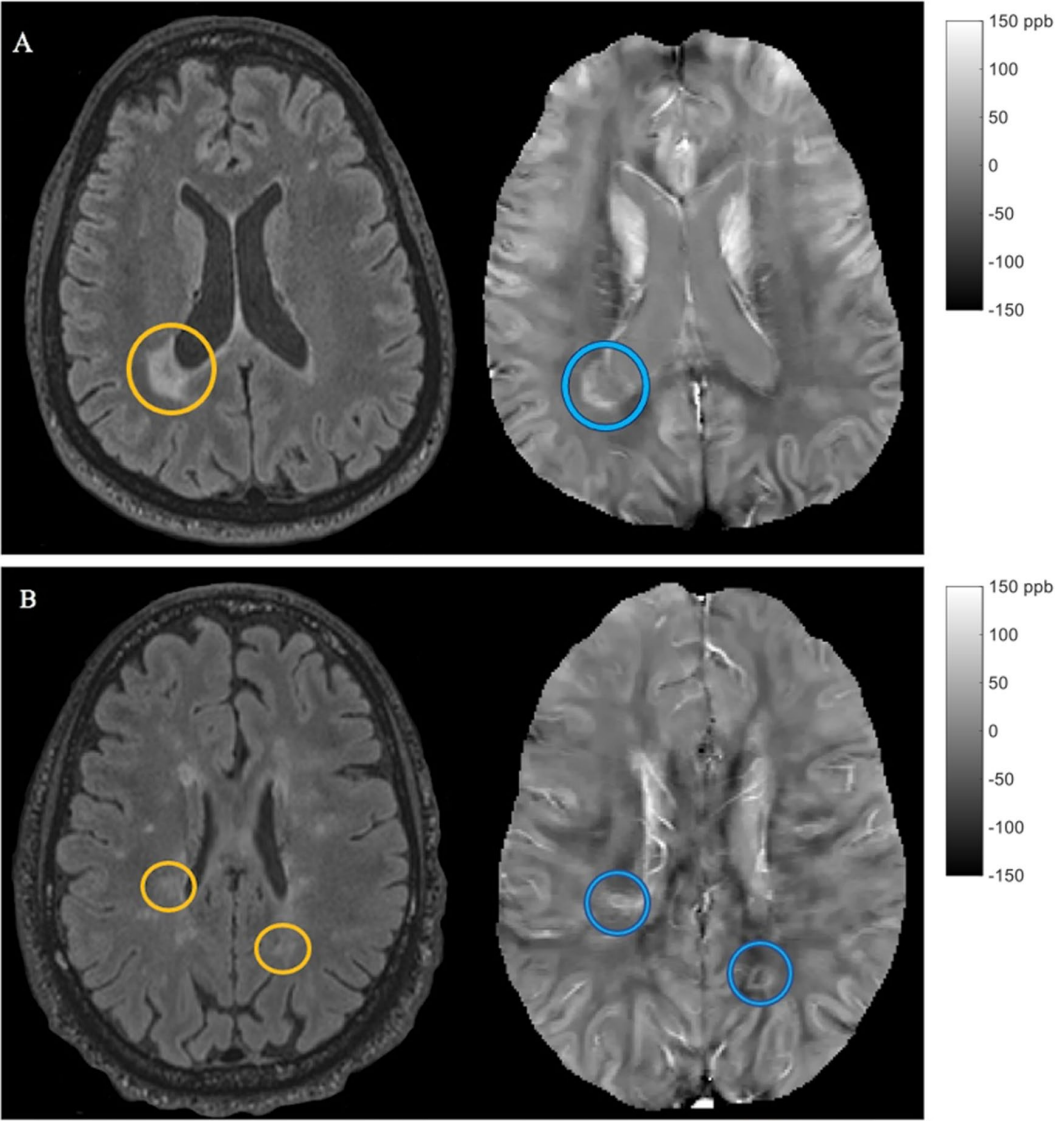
Examples of QSM rim+ lesions. Panels (A) and (B) represent slices of imaging for two separate patients. Within each panel, FLAIR is located on the left, and the corresponding slice of QSM is located on the right. In both panels (A) and (B), the lesion on FLAIR is located within an orange circle, while the corresponding lesion on QSM is located within a blue circle. These examples represent lesions with a complete rim on QSM.
